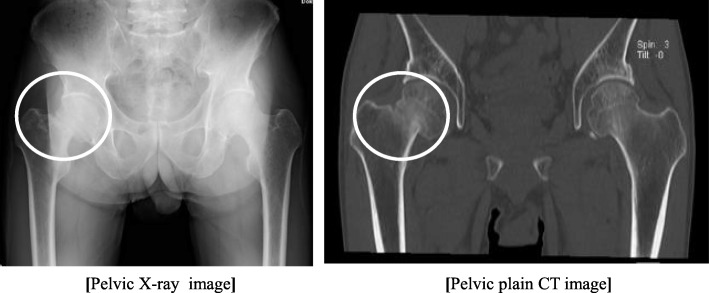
Pelvic x-ray and computed tomography. The *circles* show a fracture of the right femoral neck
